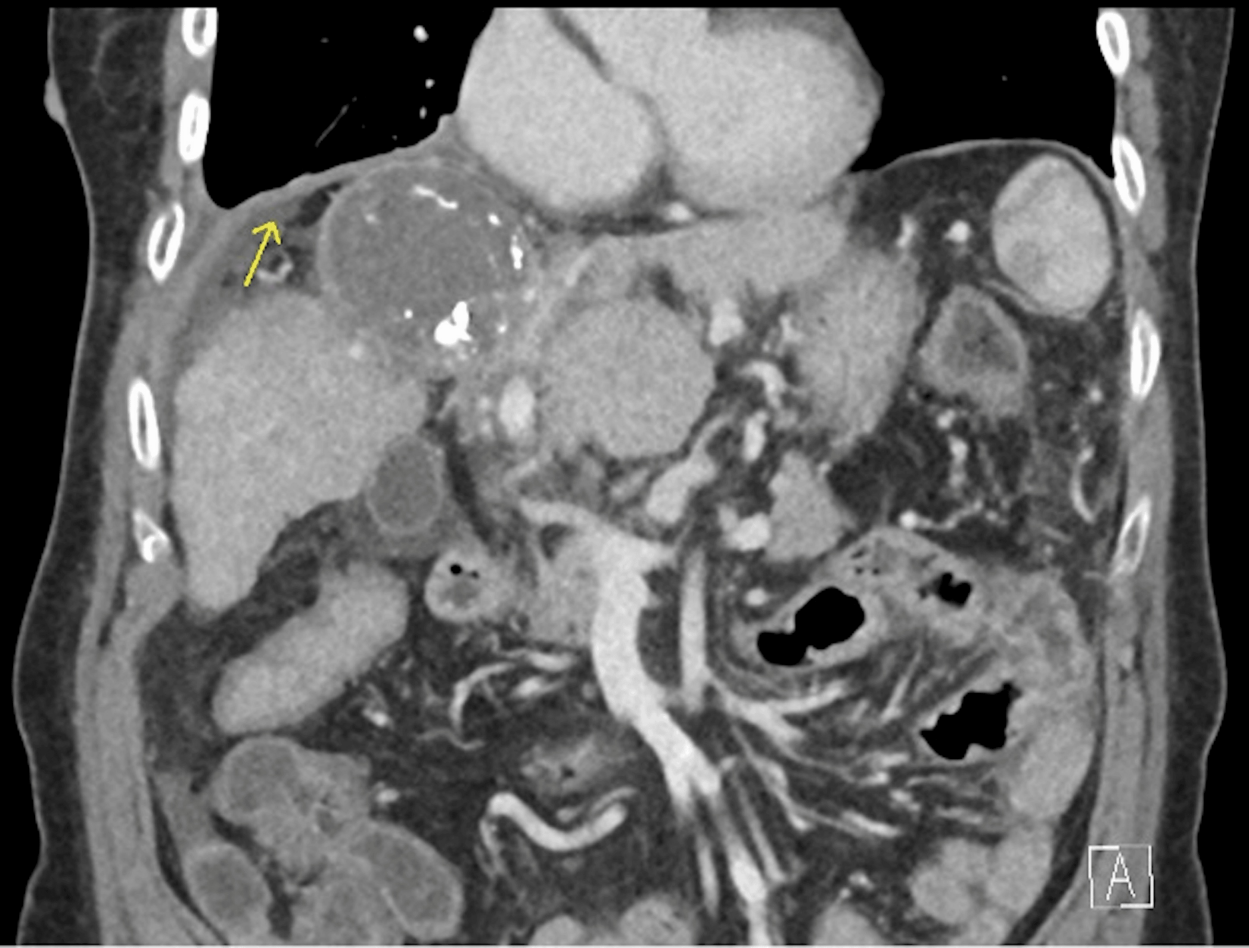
CT liver performed six weeks post-diaphragmatic hernia repair demonstrating intact right diaphragmatic repair with no recurrence of herniation.
